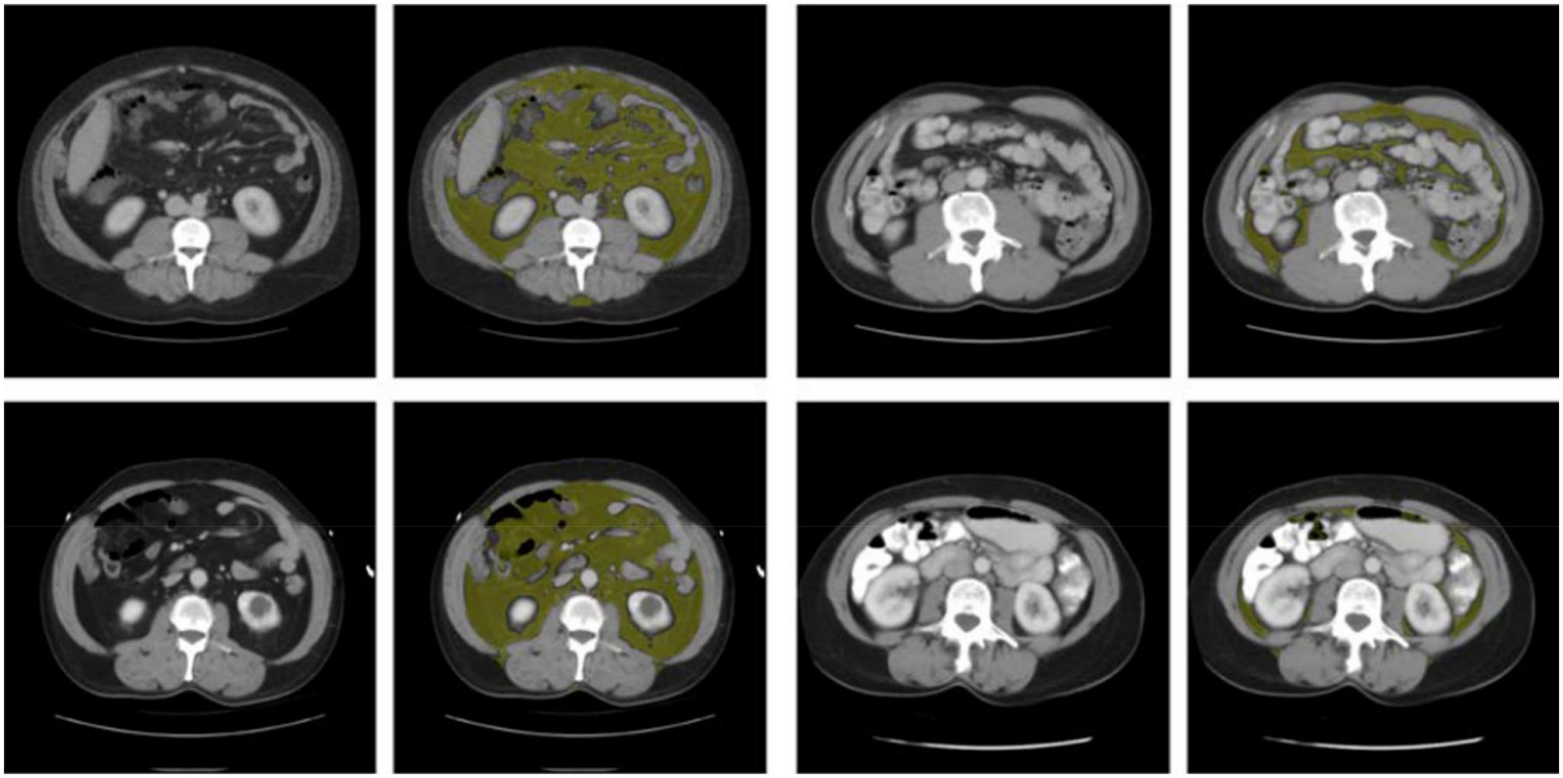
Axial CT images without (left) and with (right) depiction of the automated segmentation of visceral adipose tissue (VAT) at the L3 level in 4 patients, corresponding to high VAT area/high VAT HU (upper left), high VAT area/low VAT HU (lower left), low VAT area/high VAT HU (upper right), and low VAT area/low VAT HU (lower right) combinations.
